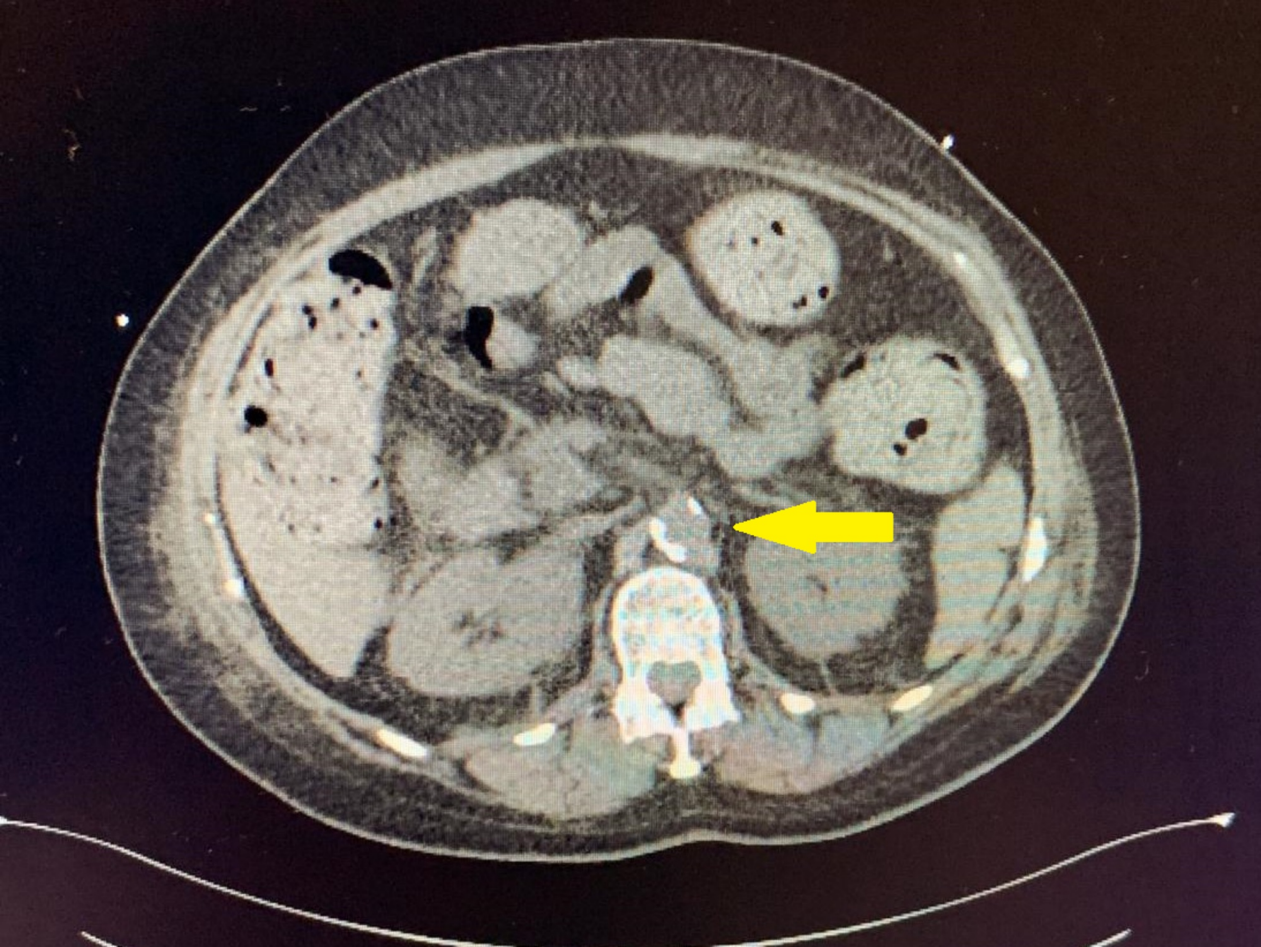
CT scan of the abdomen showing a pancreatic head lesion.
